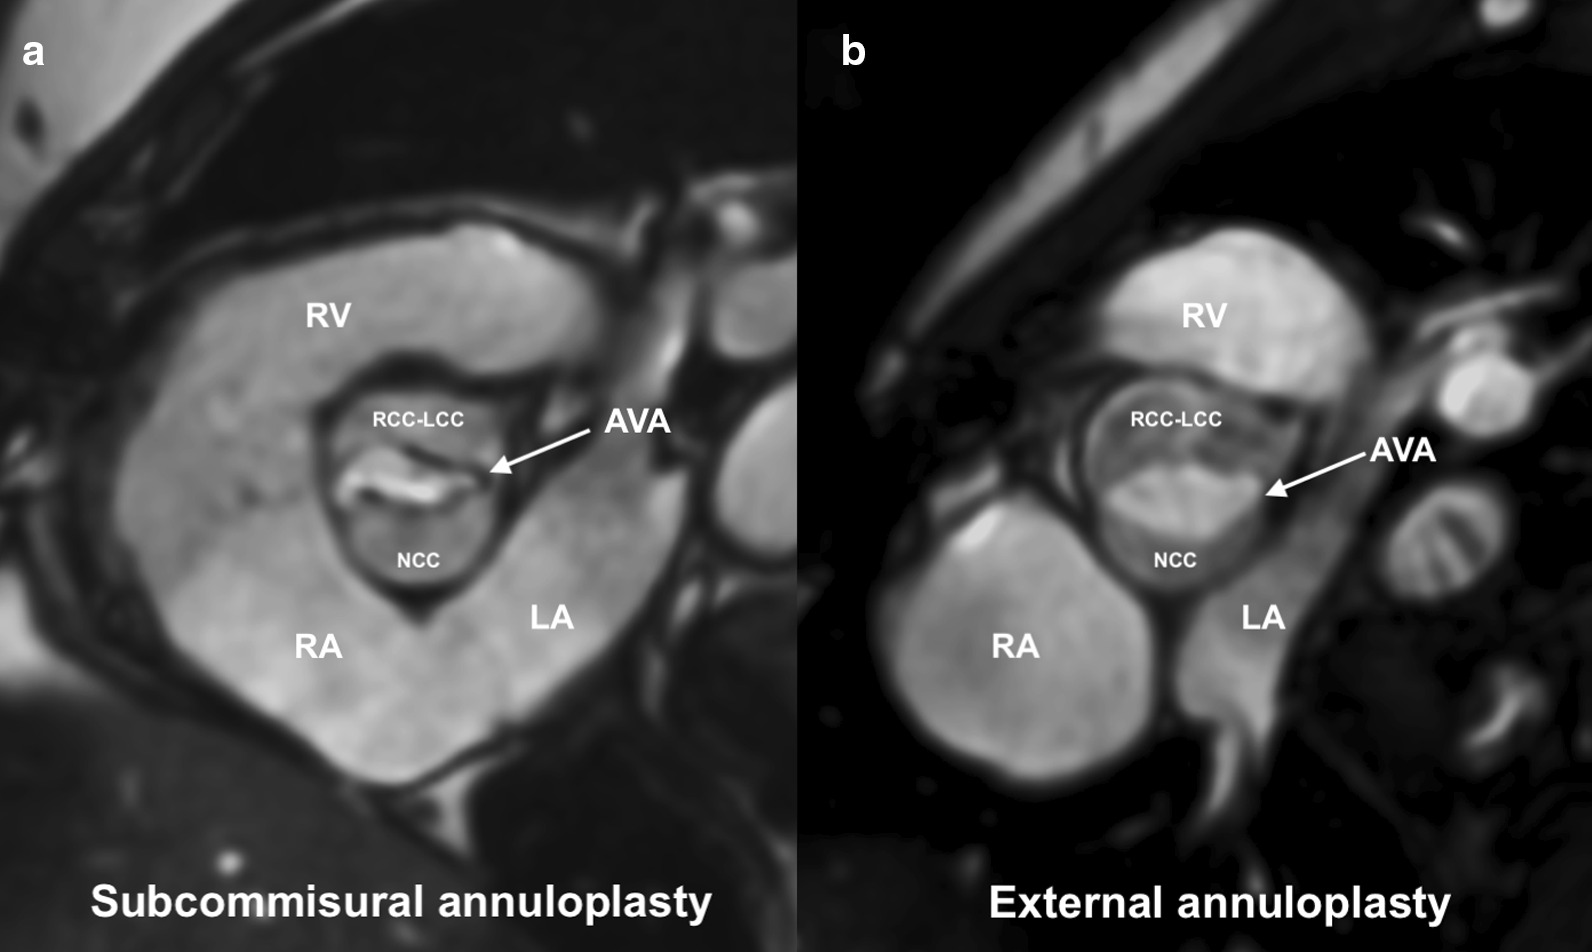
Cardiac magnetic resonance imaging of bicuspid aortic valve at end-systole in two patients treated with subcommissural annuloplasty (a) and external annuloplasty (b). After external annuloplasty (b) larger aortic valve area (AVA) was associated with lower transvalvular gradients as compared with subcommissural annuloplasty (a). AVA, aortic valve area; LA, left atrium; LCC, left coronary cusp; LV, left ventricle; NCC, noncoronary cusp; RA, right atrium; RCC, right coronary cusp; RV, right ventricle
